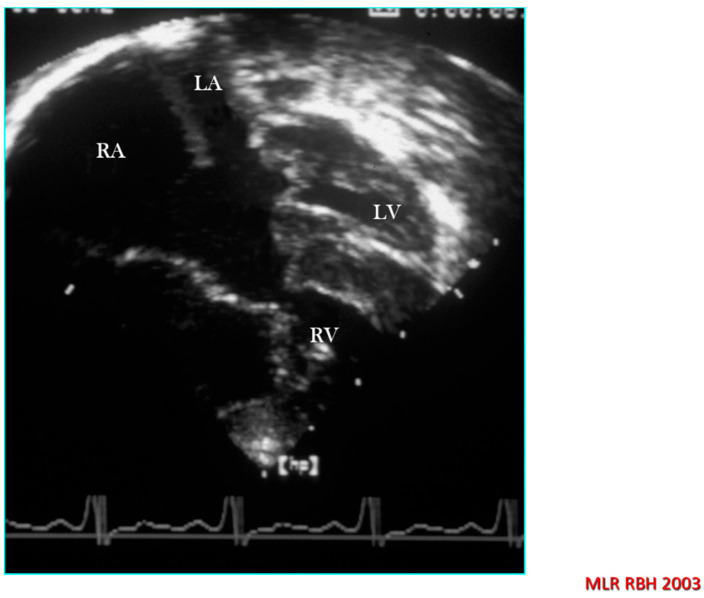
Echocardiographic four chamber section from a heart with partial AVSD, hypoplastic left ventricle and miniaturised left atrioventricular valve orifice.
